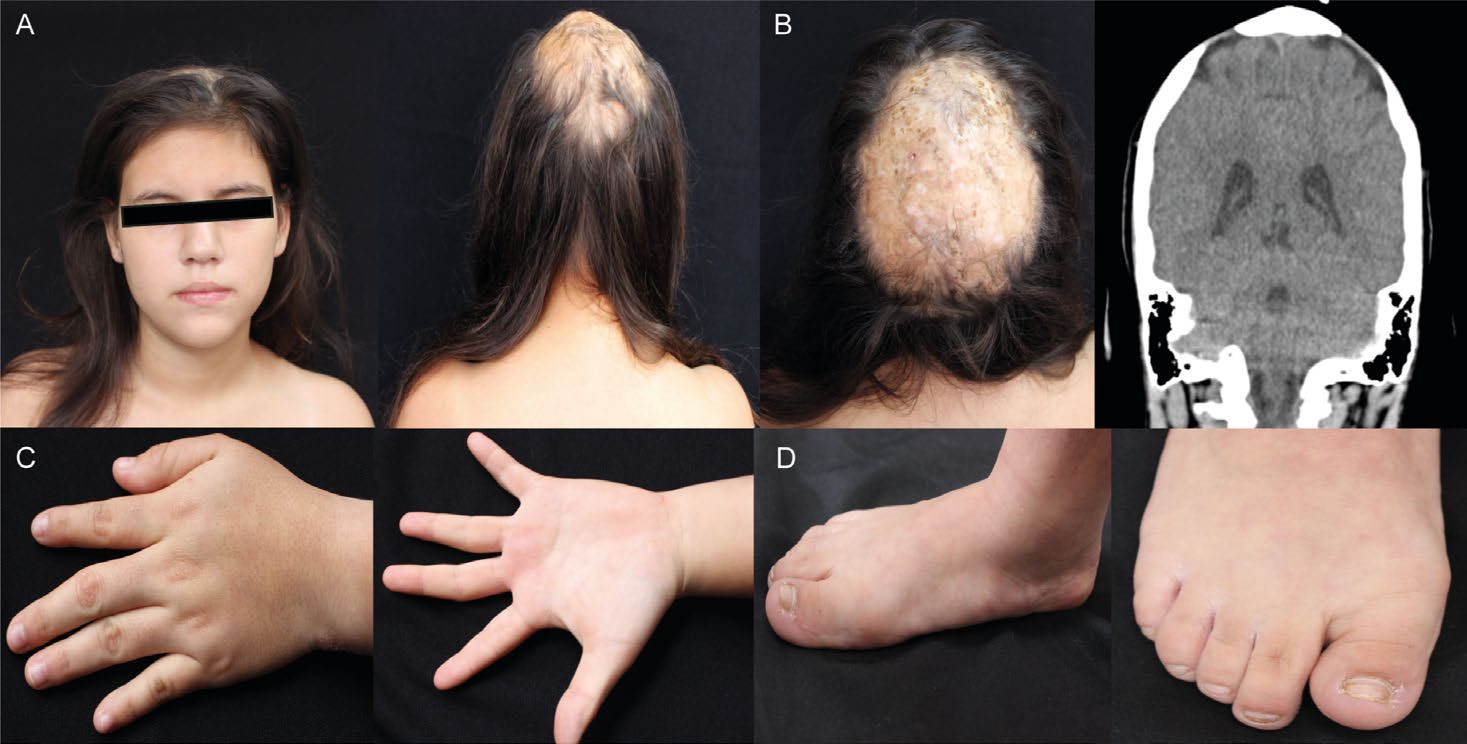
Adams–Oliver type 6 Patient: (A) Front and back: Fitzpatrick phototype III patient with dolichocephaly and craniosynostosis. (B) Skin and skull: Scalp with atrophic and alopecic areas, and CT scan showing bitemporal bone anomalies. (C) Left hand: Mild syndactyly (3rd and 4th fingers) and symbrachydactyly. (D) Right toe: Hypoplastic nails.
